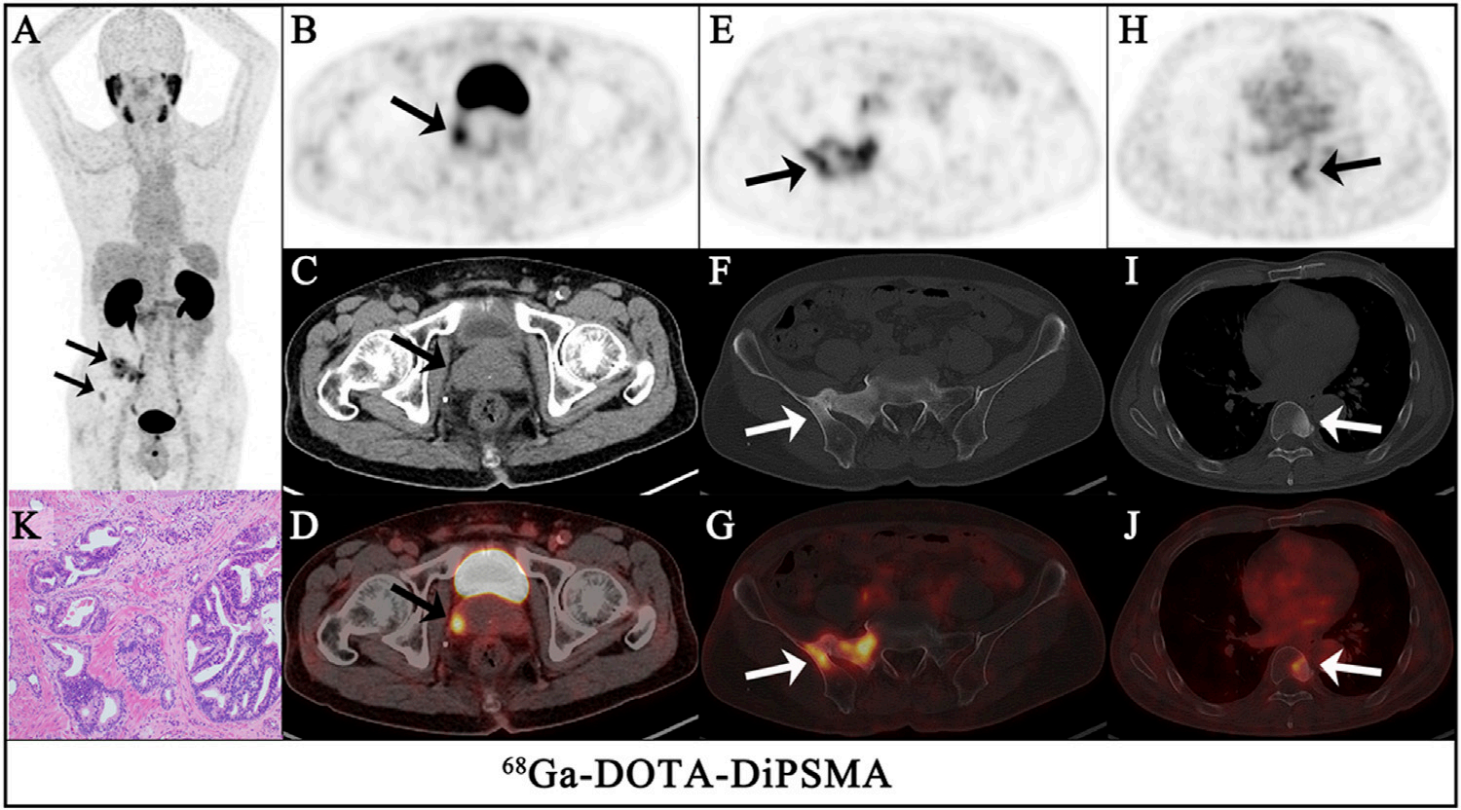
A 68-year-old-man had confirmed prostate cancer after a needle biopsy of the prostate for 1 week. The maximum intensity projection (MIP) of ^68^Ga-DOTA-DiPSMA PET/CT [(A), arrows] showed significantly abnormal uptake in the image. Axial views of the prostate (top, PET; middle, CT; bottom, fusion image) show intense uptake (SUV_max_ 3.3) in the isodense nodule of the prostate [(B–D), arrows]. In the other level axial views, increased ^68^Ga-DOTA-DiPSMA uptake was observed in the sacrum and iliac [(E–G), arrows] and the 8th thoracic vertebrae lesions [(H–J), arrows], which were concomitant with bone density increased. Postoperative pathology confirmed it as adenocarcinoma of the prostate (K).
